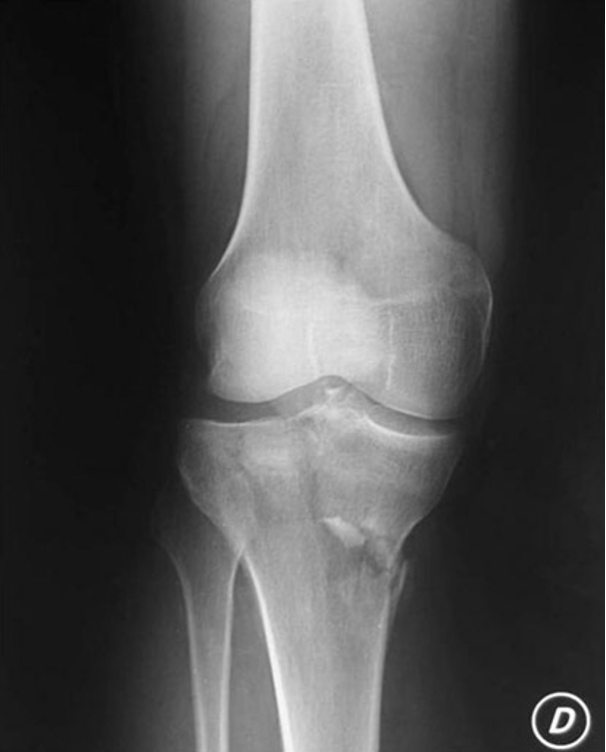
Schatzker type IV fracture, pre-op X-rays (AP)
